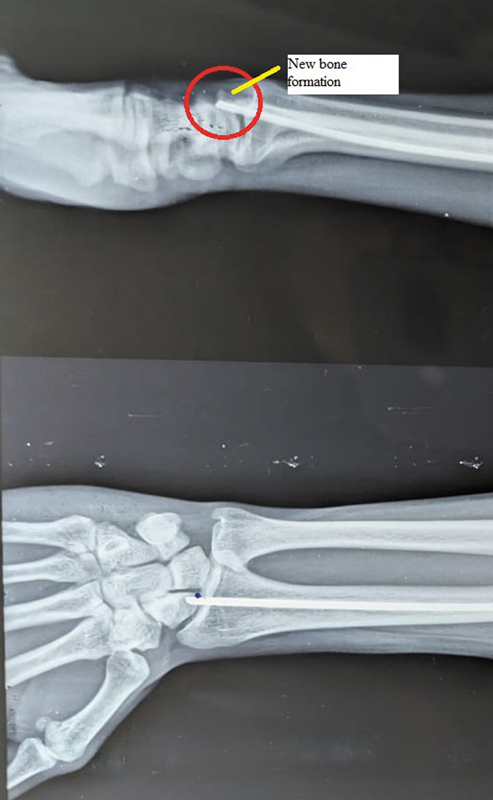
Preoperative radiographs of the right hand and distal forearm showing intramedullary titanium elastic nail system (TENS) nail impinging the extensor compartment.
